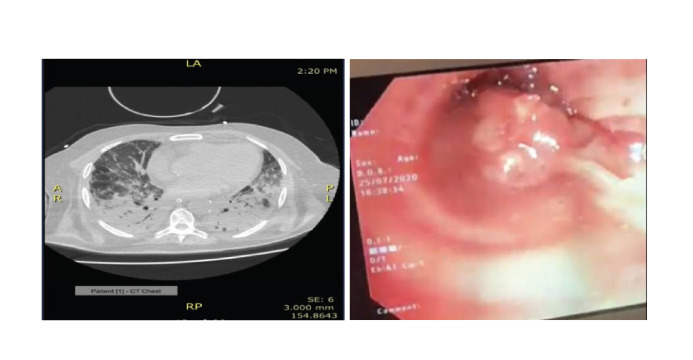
Computed tomography scan (A) for patient 1 and (B) fibrinous plugs in lobar bronchi.
